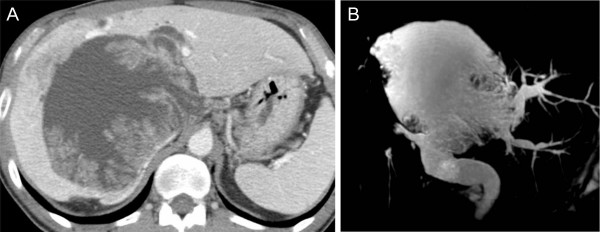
Axial image of enhanced computed tomography shows a bulky cystic mass with multiple internal papillary projections involving the right hemiliver and left medial section (A). Magnetic resonance cholangiography (MRC) reveals a marked aneurysmal dilatation of the bile duct itself of the left medial section and a diffuse dilatation of the extrahepatic bile duct (B).
